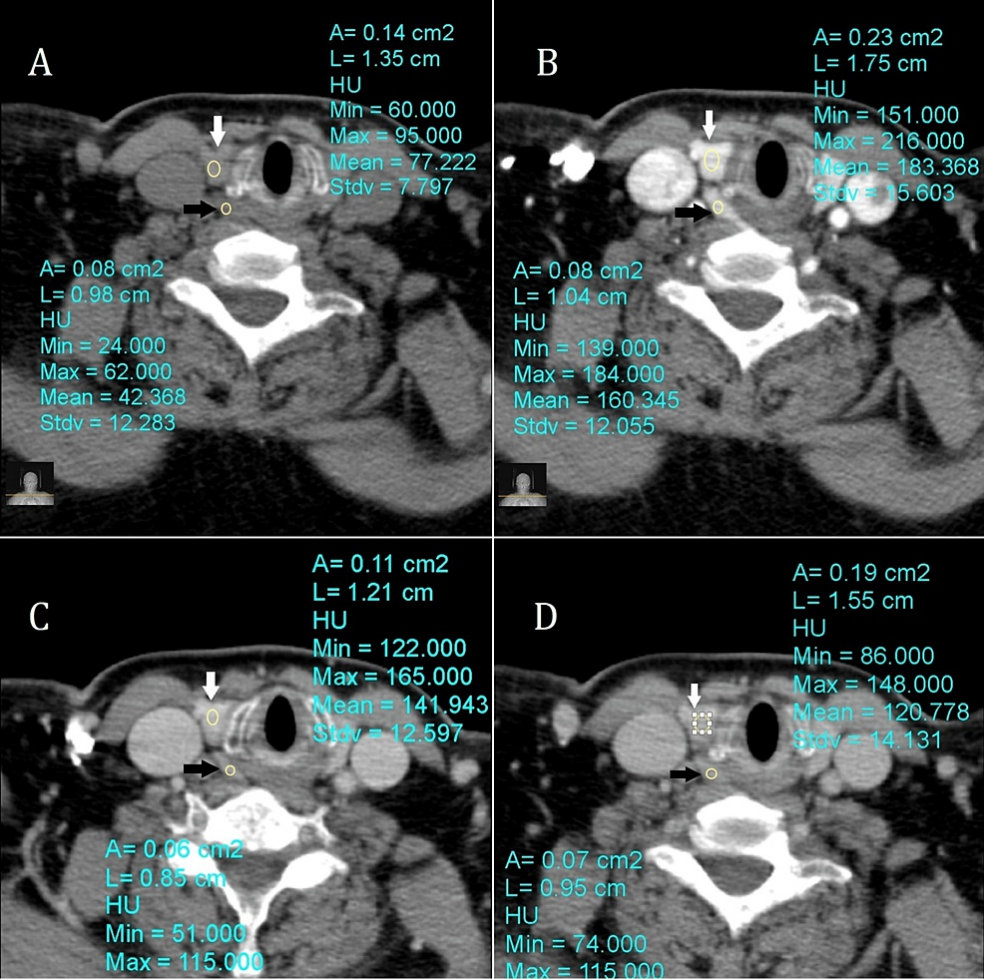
Demonstration of measurement technique on the axial images of the 4-dimensional CT scan. Axial images on the non-enhanced (A), arterial (B), venous (C), and delayed (D) phases at the level of the thyroid gland. In the precontrast scan (A), note the significantly higher attenuation measured by ROI (yellow circle) of the thyroid gland (white arrow) as compared to the parathyroid adenoma (black arrow). The parathyroid adenoma (black arrow) as well as the thyroid gland (white arrow) demonstrate the highest attenuation in the arterial phase (B). In the venous (C) and delayed (D) phases, note the rapid washout of the contrast from the parathyroid adenoma (black arrow).
